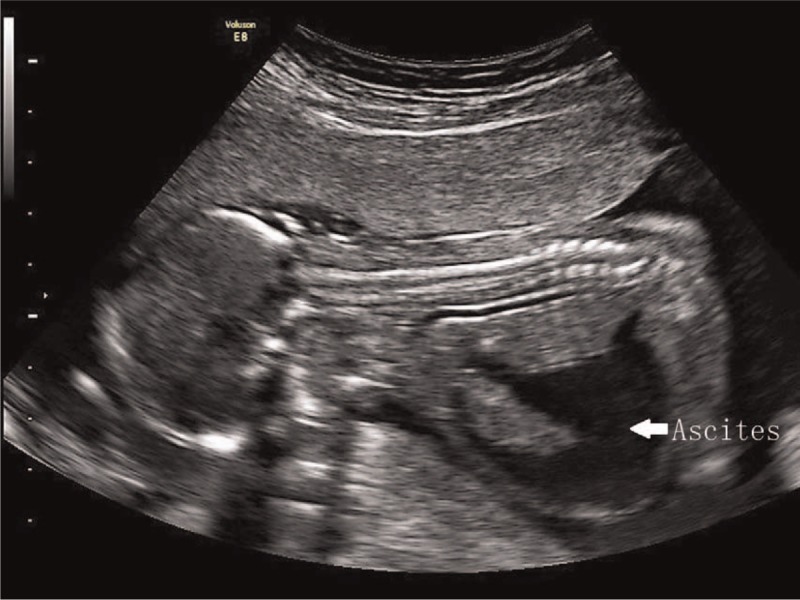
The sonographic examination of case 2 at 16 weeks’ gestation. No structural abnormalities were found, but unexplained ascites (white arrowhead) and thickened nuchal fold were identified.
